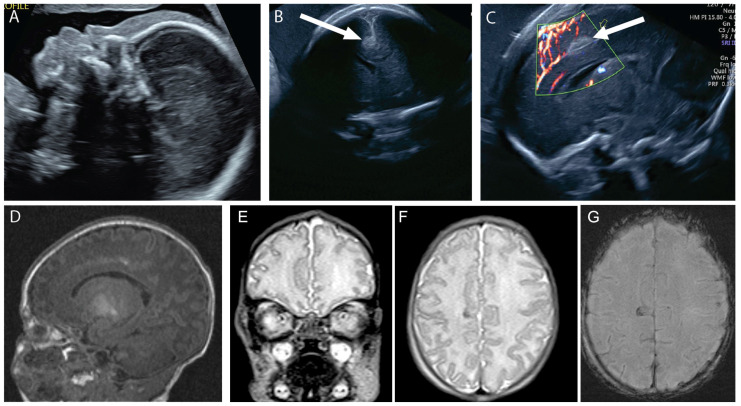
A 20-year-old G1P0 was referred for fetal midface hypoplasia (A). Neurosonogram at 25 weeks revealed a focal cortical abnormality in the right mesial cortex superior to the corpus callosum and just to the right of the falx (arrows, (B,C)). The patient’s cfDNA results were normal, and the mother declined amniocentesis. A fetal MRI at 26 weeks 2 days’ gestation revealed similar findings of mid-face hypoplasia and possible right medial cortical maldevelopment. Repeat MRI at 32 weeks’ gestation revealed similar findings (D). The baby was delivered vaginally at 37 weeks 2 days’ gestation and was admitted to the NICU. Postnatal MRI showed focal cortical abnormality in the mesial aspect of the right frontal lobe (E–G). The progression of the echogenic finding in the mesial cortical region is compatible with thrombosis of a superficial mesial cerebral vein, which is frequently associated with necrosis and gliosis of the adjacent cortical area, which can be seen in the T2 signal drop and the associated susceptibility artifact on susceptibility weighted imaging. The child’s SNP array was normal male. At one year of age, the child was undergoing physical and occupational therapy for a missed developmental milestone. By two years of age, the child was meeting milestones but had significant behavioral problems.
